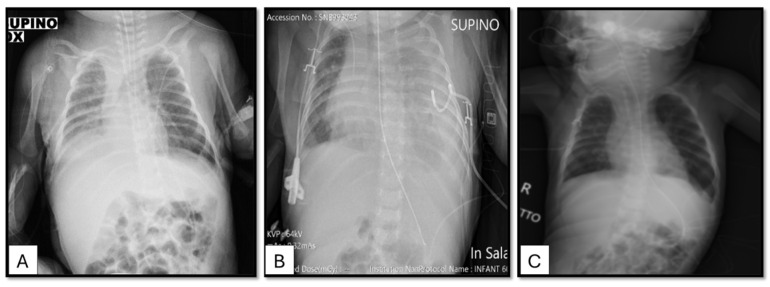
Evaluation of chylothorax of the proband by chest X-rays before and after treatment with trametinib. (A) Patient on the second day of life: right pleural effusion. (B) Patient after octreotide therapy: worsening of the pleural effusion. (C) Patient after 24 days of trametinib therapy: absence of chylothorax.
